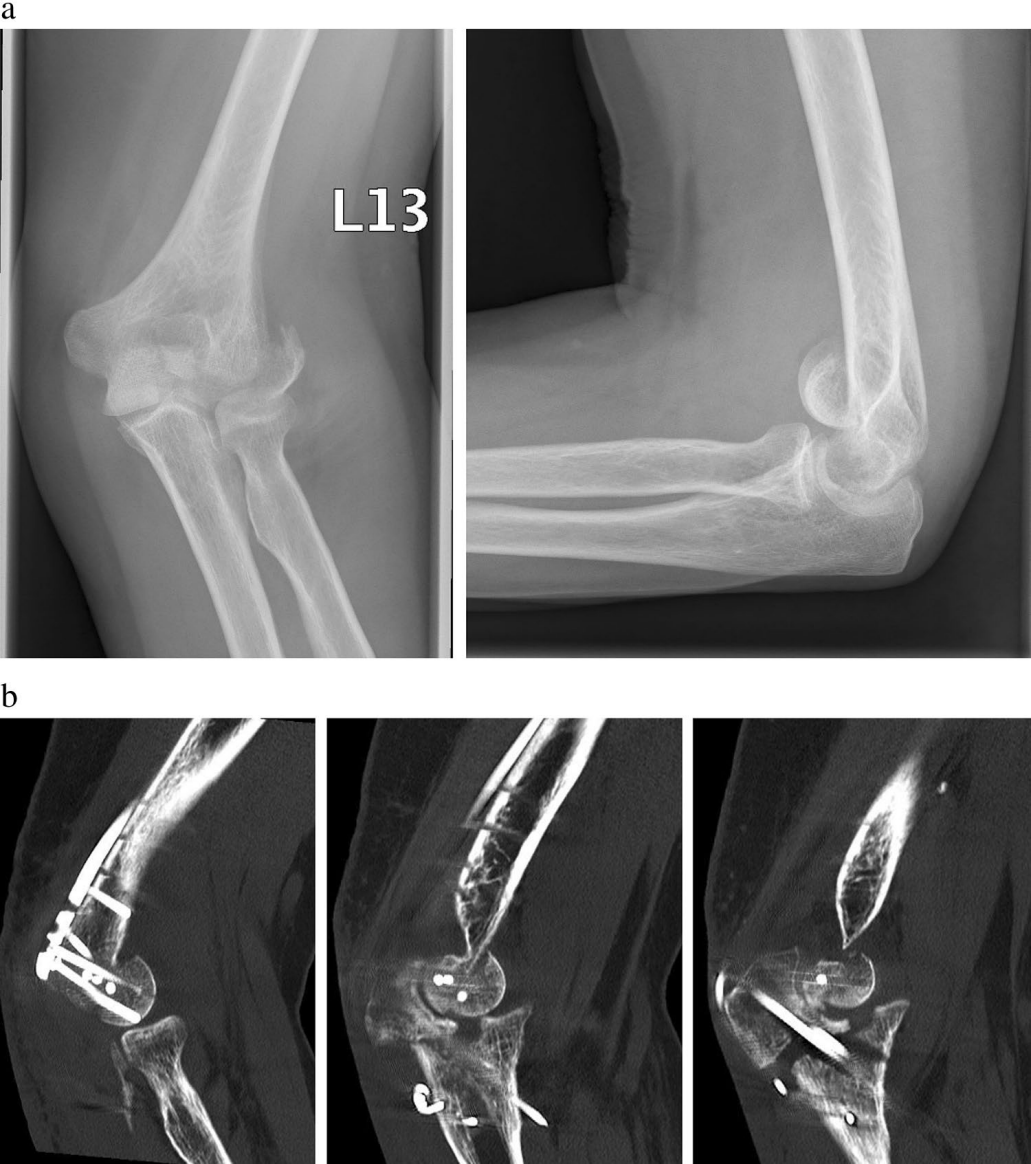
Case of a 63-year old female patient with a left-sided coronal shear fracture of the distal humerus (Dubberley type 3A) with concomitant fracture of the lateral condyle. She underwent ORIF with cannulated screws and a posterolateral distal humerus plate through a trans-olecranon approach. The patient presented to our office for the first time two months postoperatively due to limited range of motion of the affected elbow and sensory ulnar neuropathy. A Plain radiographs of the left elbow in an anteroposterior and lateral view following trauma show a displaced Dubberley type 3A fracture. B A CT scan of the left elbow two months postoperatively reveals insufficient reduction of the articular surface of the distal humerus. The olecranon osteotomy was performed too distal and significant incongruity of the articular surface of the proximal ulna remained following refixation with a tension band
